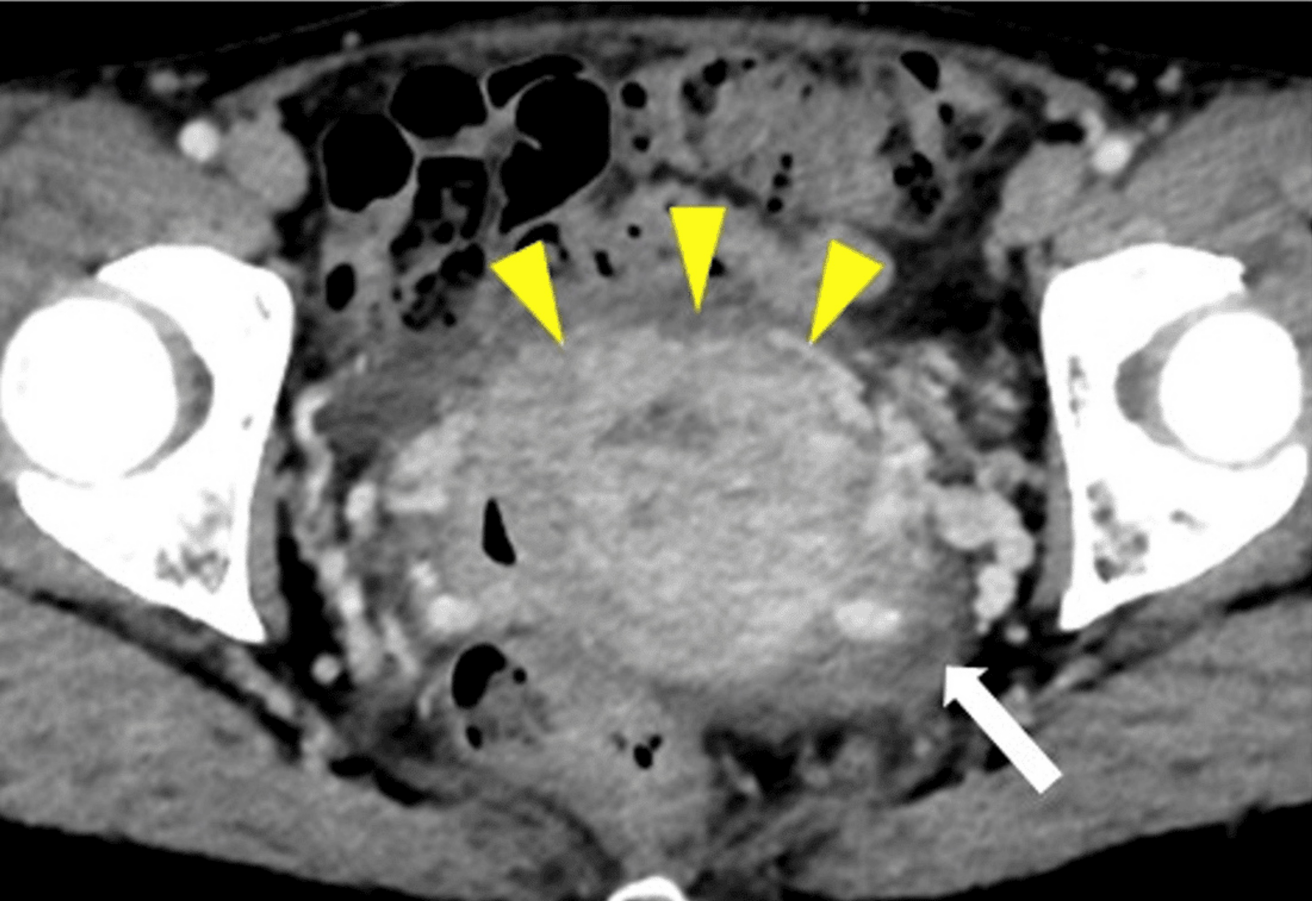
Computed tomography (CT) findings. The contrast-enhanced CT shows cervical swelling. The yellow arrowhead indicates cervical swelling. The white arrow indicates lymph node enlargement.
